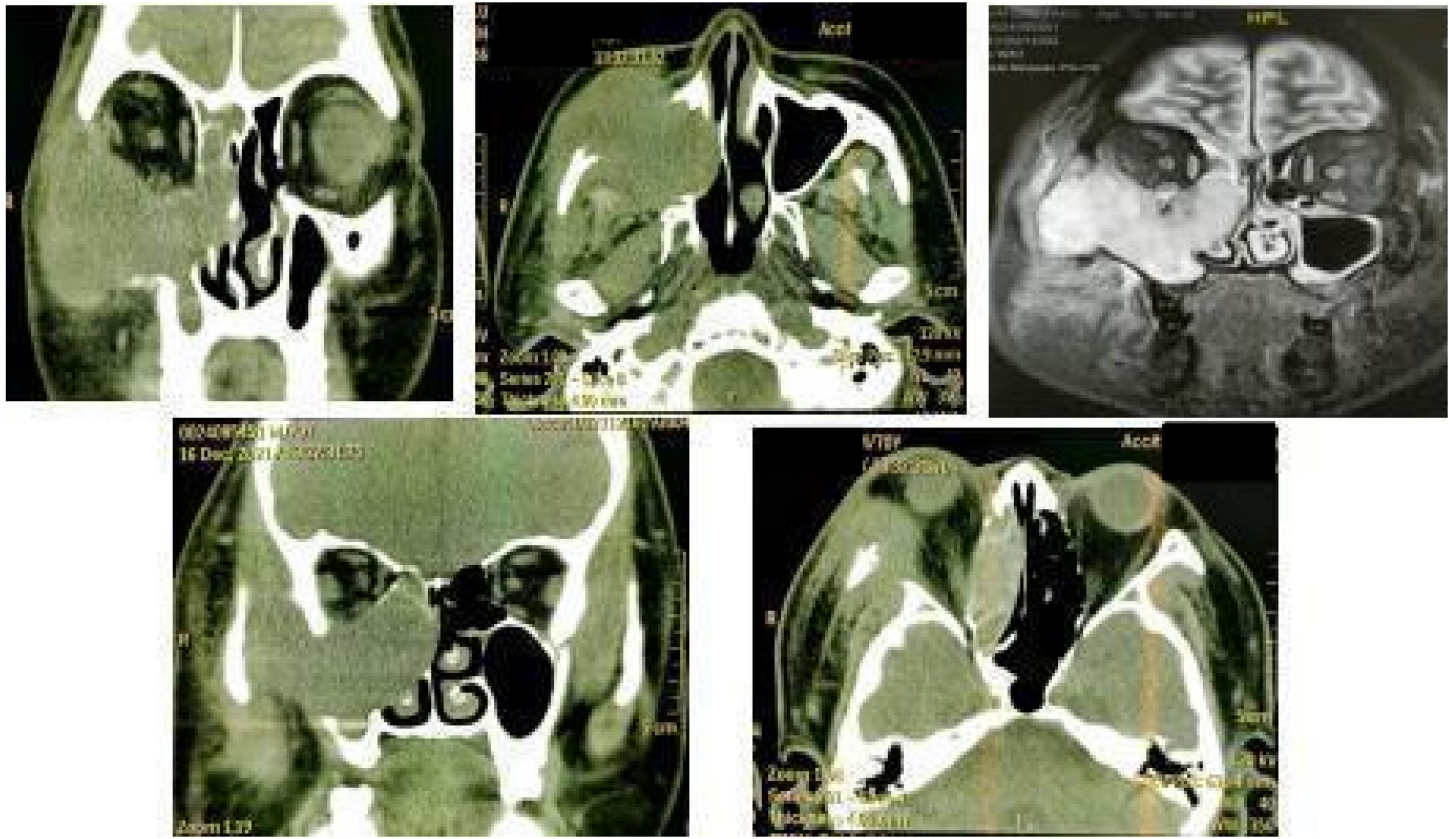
3D-CT and MRI images of the space-occupying lesion in the right maxillary sinus.
